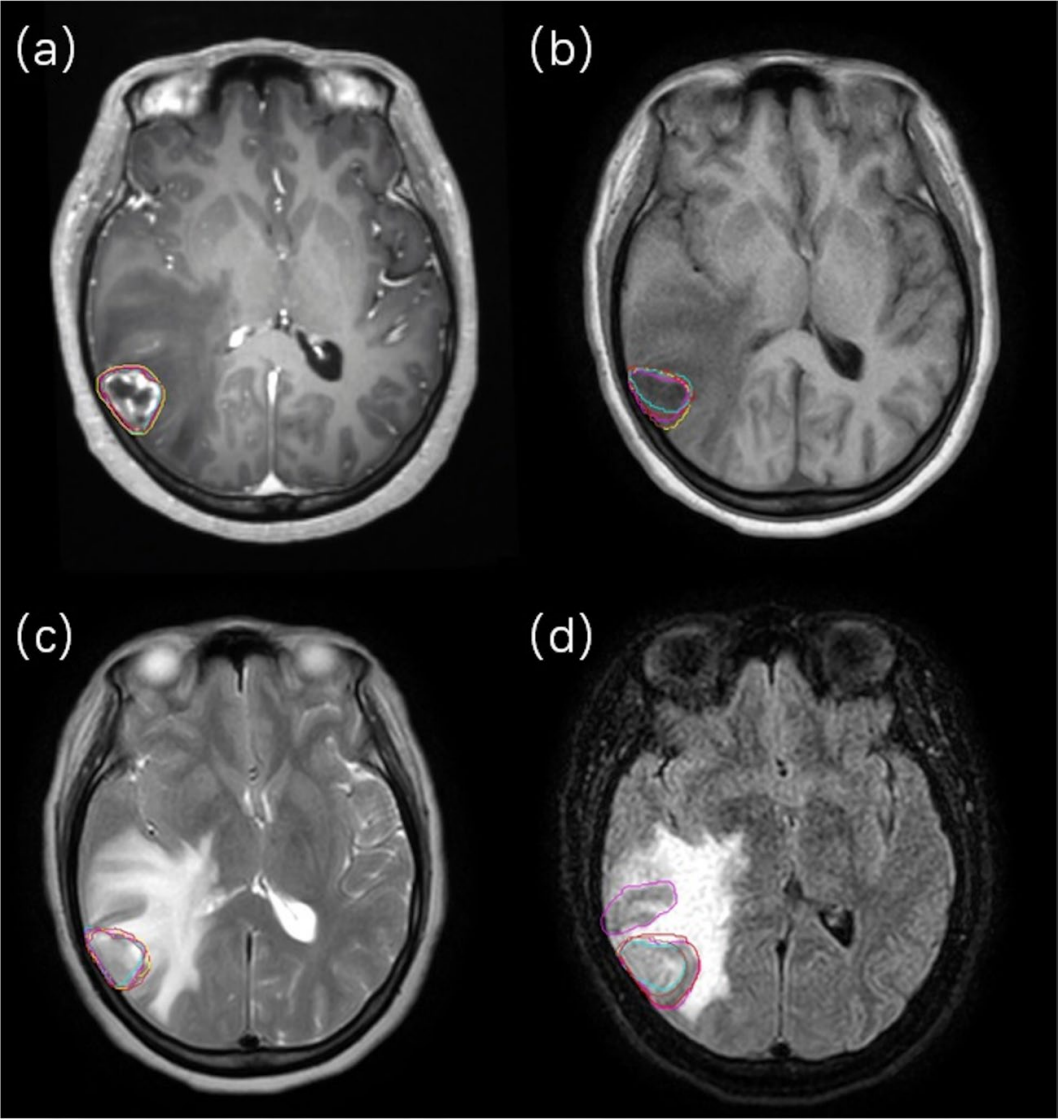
A case with a single brain metastasis (BM) larger than 3 cm^3^ as shown in different MR images (a) pre-treatment T1c, b online-T1, c online-T2, d online-FLAIR, and contours of different observers (lines with different colours)
